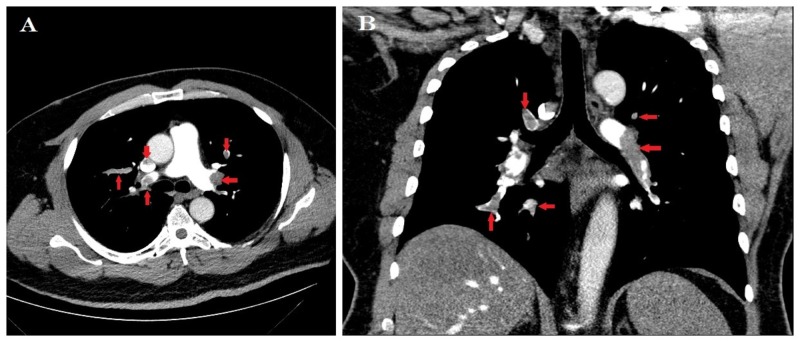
Axial (A) and sagittal (B) contrast-enhanced computed tomography pulmonary angiogram images demonstrating multiple bilateral filling defects (arrows) suggestive of pulmonary emboli
